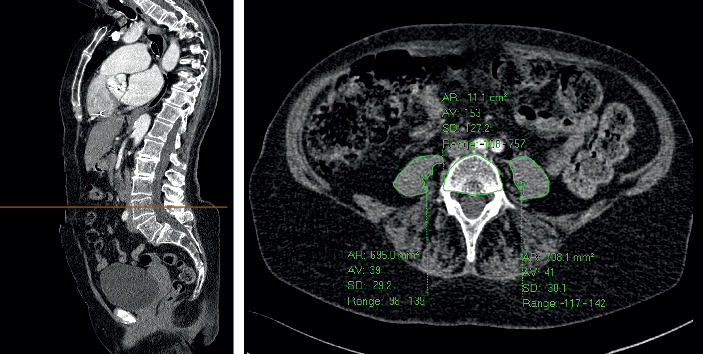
Cross-sectional psoas and vertebral body areas derived from an axial CT image at the level of L4, using a freehand region-of-interest tool (green outlines). In this patient, the PLVI was mean psoas area (mm^2^)/vertebral body area (mm^2^) ((695 + 708)/2)/1110 = 0.63.
